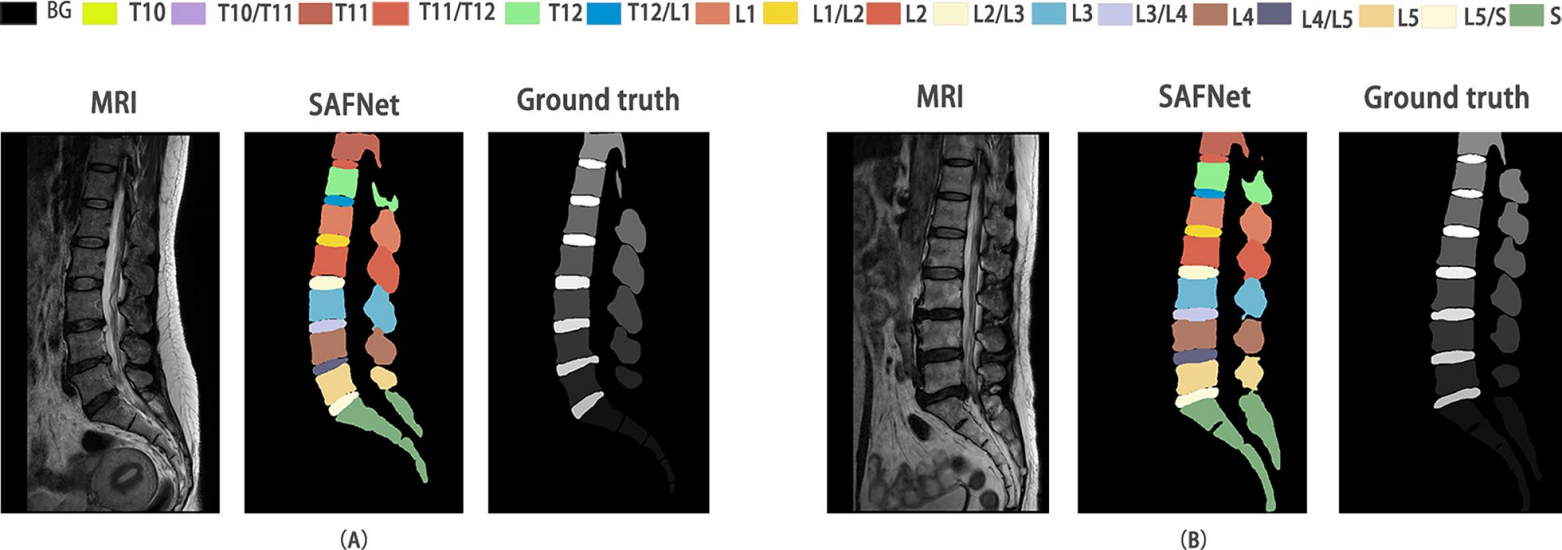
SAFNet segmentation MRI vertebral results. A and B are the results of the spinal mid-sagittal plane segmentation for six subjects using SAFNet. (BG represents the background; MRI denotes magnetic resonance imaging; SAFNet denotes scene aware fusion network; DSC denotes Dice Similarity Coefficient.)
